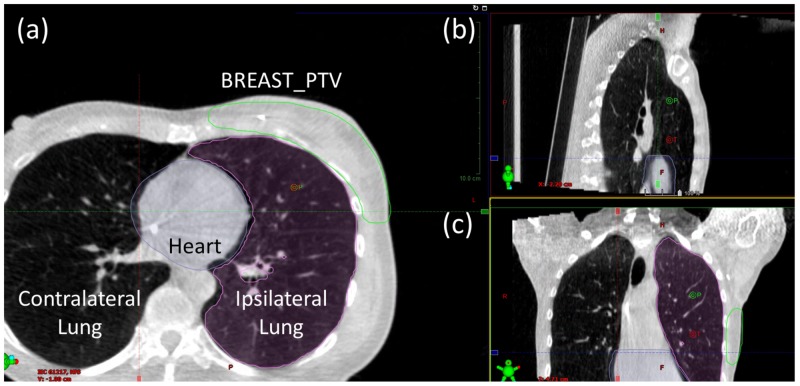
Breast protocol CBCT of tangent fields shown in the (a) axial, (b) sagittal, and (c) frontal views Overlays of the heart (purple), ipsilateral lung (pink), and BREAST_PTV (green) structures from the planning CT allow assessment of alignment quality. CBCT, cone-beam computed tomography
